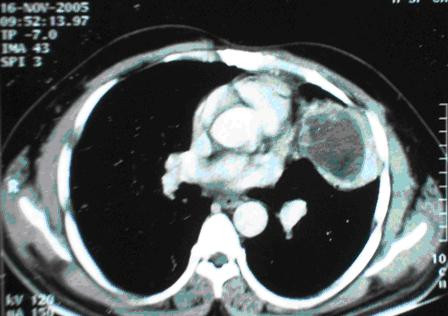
Computed tomography (CT) of the thorax showed a well defined lesion in the anterior segment of the left upper lobe.
